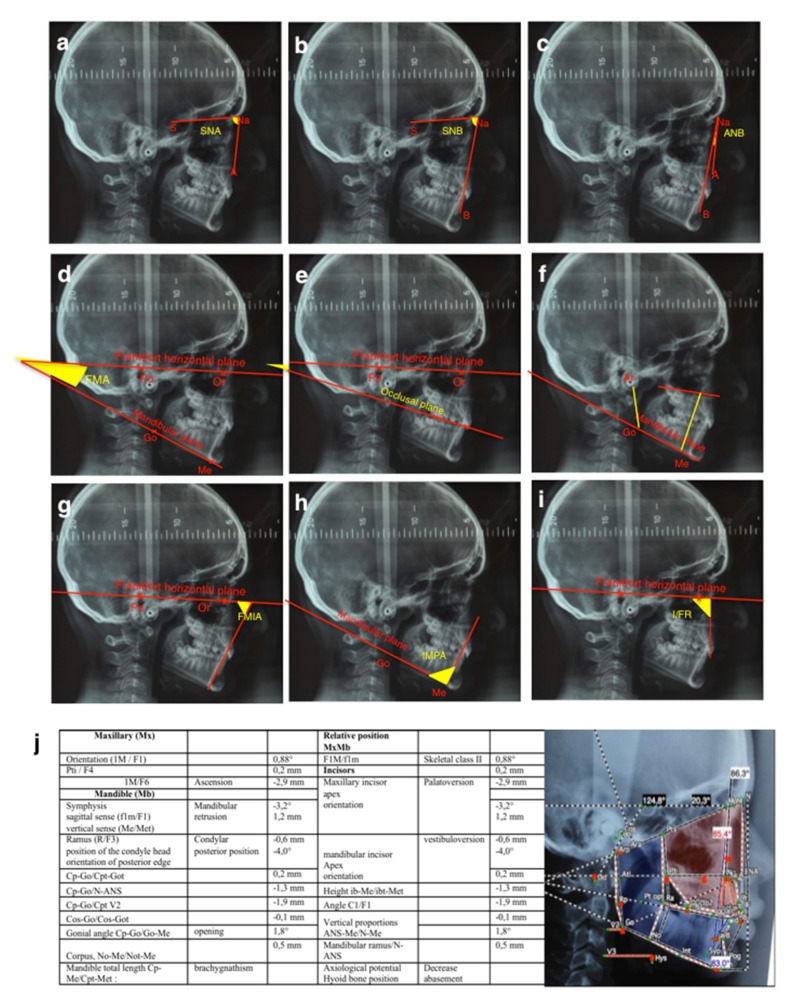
Cephalometric landmarks of Tweed’s (a-i) and Delaire’s (j) analyses. For Tweed’s analysis, the antero-posterior skeletal measurements were (a) the SNA, corresponding to the antero-posterior position of the maxilla in relation to the anterior cranial base, (b) the SNB, corresponding to the antero-posterior position of the mandible in relation to the anterior cranial base, and (c) the ANB, corresponding to the discrepancy between the maxilla and the mandible. For the vertical skeletal measurements, the FMA (d) corresponds to the Frankfort mandibular plane angle. (e) and (f) present respectively the occlusal plane/Frankfort angle and the vertical index measurements. (Ar: Articular point; Go: Gonion; Me: Menton). Concerning the dental measurements, (g) FMIA corresponds to the Frankfort mandibular incisor angle, (h) IMPA to the incisor mandibular plane angle and (i) I/FR to the upper incisor/Frankfort angle. For Delaire’s cephalometric analysis, (j) the table summarizes the different measurements made, as presented on the annotated tele-X-rays based on the positions of the following referent points: M, Metanasion; F1, anterior craniofacial balance; F4, craniopalatal point; C1, craniofacial base line; F, frontal; Op, posterior occipital point; Clp, posterior clinoid process; CT, temporal condylar point; Pts, superior pterygoid point; Na, nasion projected; ANS, anterior nasal spine; Go, gonion; FM, frontomaxillary articulation; Cp, posterior tangent of the condyle; Pti, inferior pterygoid point; NPC, naso-palatine canal; Me, menton; Bo, Bolton point; Od, odontoid; Om, mandibular-occipital point.
